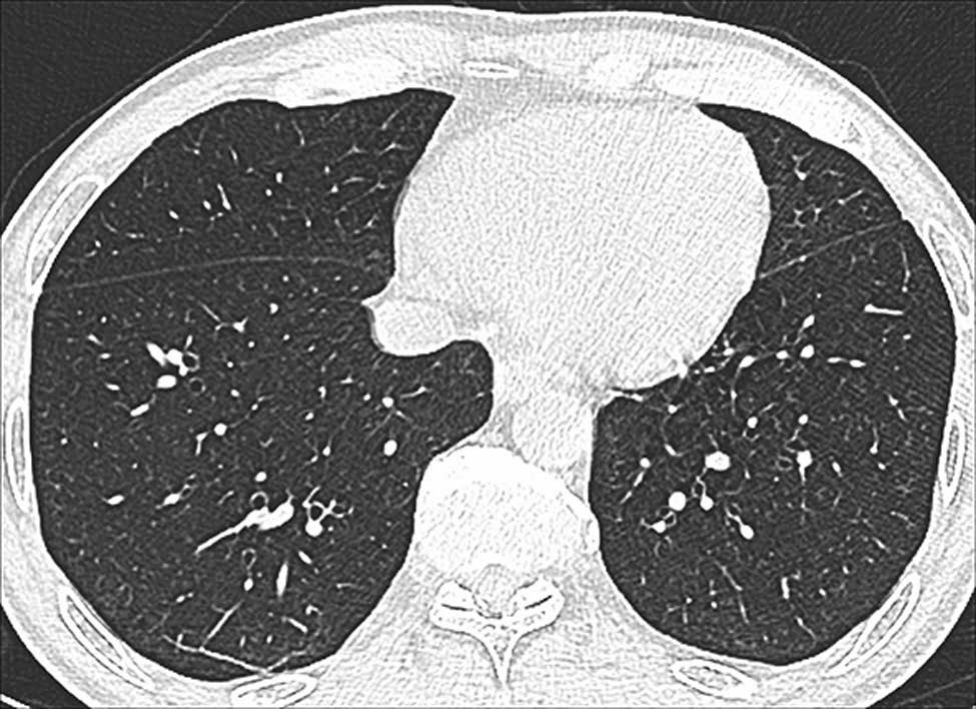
Representative CT images of SL. M, 53 yr, black arrow— subpleural line of lower lobe of right lung. CT = computed tomography, SL = subpleural line.
